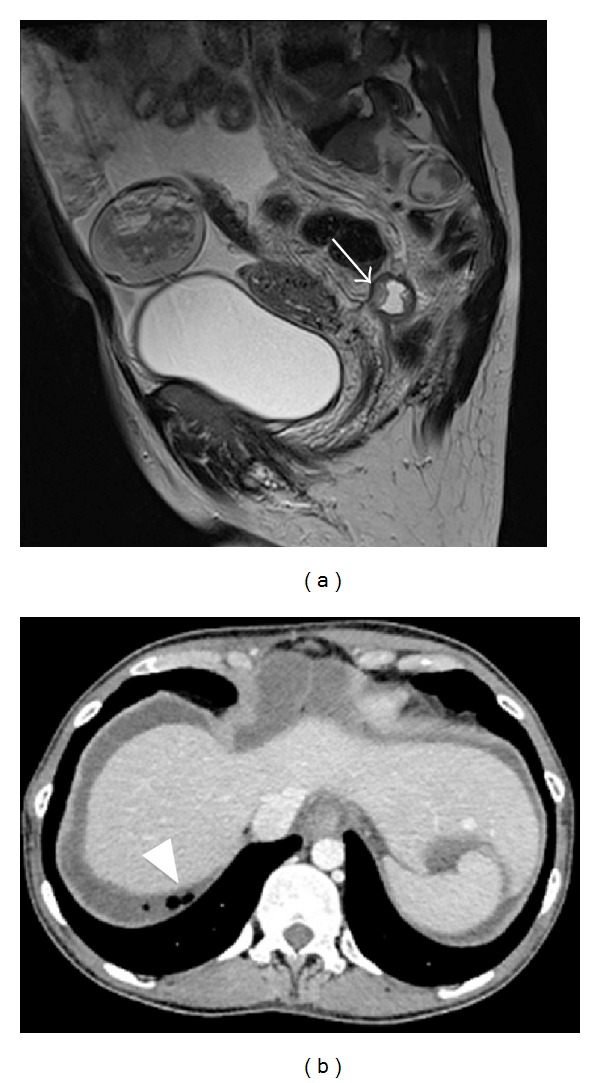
Sagittal T2 weighted image (a) and axial CT (b) demonstrate intraperitoneal rupture with fat globules in the cul de sac (arrow) and below the right hemidiaphragm (arrowhead), a pathognomonic finding.
